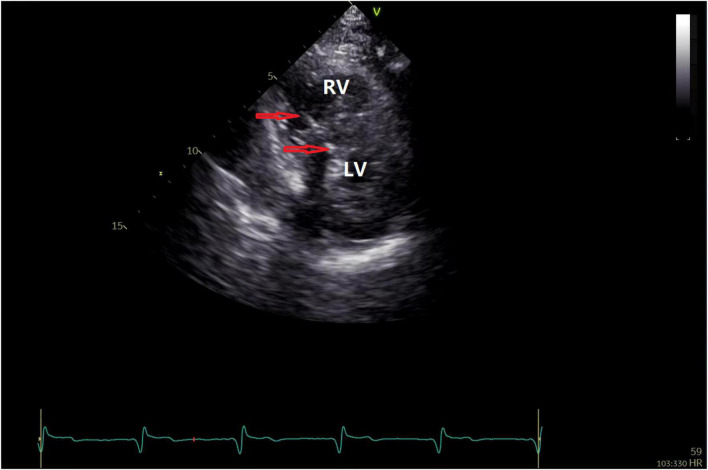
Echocardiography to position the HPSAP electrode. The red arrow indicates that the end of the pacemaker electrode is located below the endocardium of the left ventricle.
